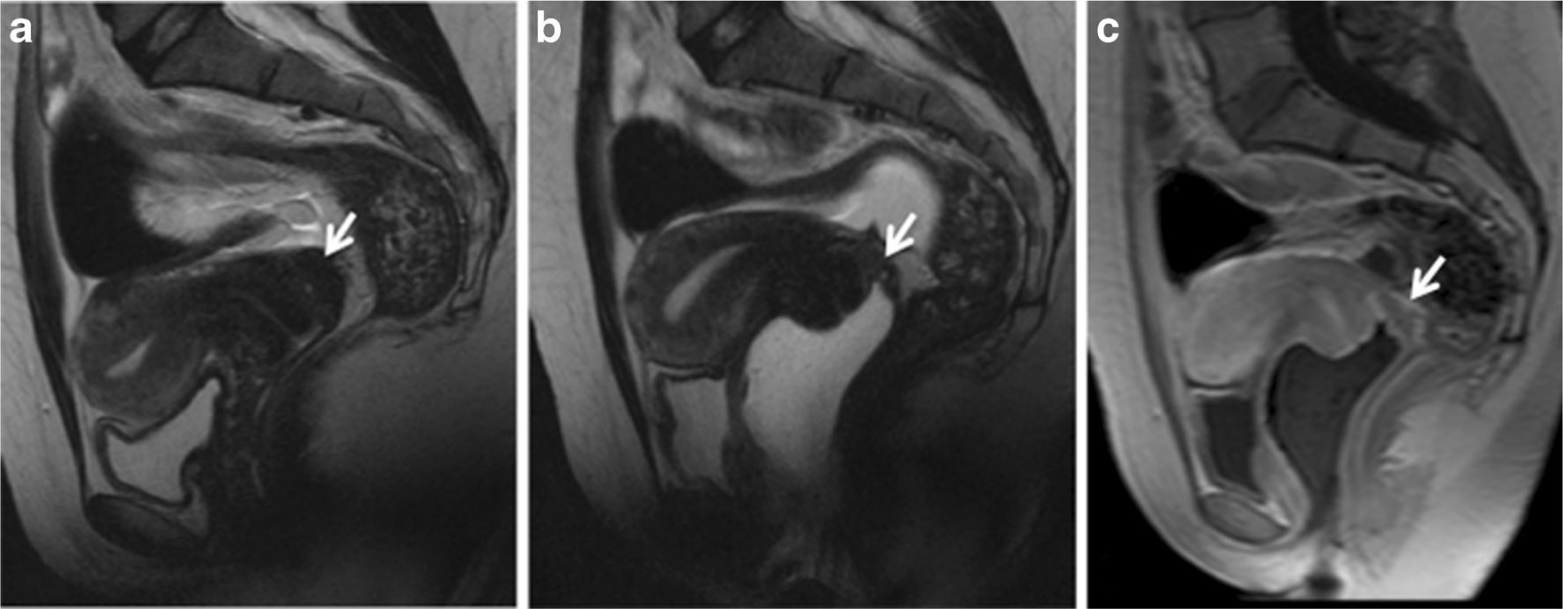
Sagittal 2D MR images performed at 1.5 Tesla demonstrating the use of sonographic gel to opacify and distend the vagina. (a) Sagittal 2D T2-weighted image demonstrating an endometriotic plaque involving the posterior vaginal fornix (white arrow). Following distension of the vagina with sonographic gel, the plaque is better delineated on both T2-weighted (b) and fat-suppressed T1-weighted (c) sequences (white arrows) (reprinted with permission - Bazot M. Ed. Lavoisier-Paris)
